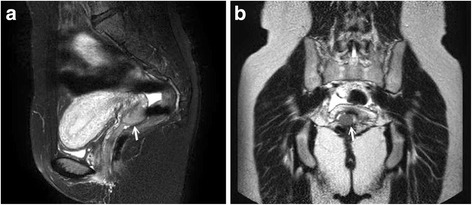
Pelvic magnetic resonance imaging findings prior to neoadjuvant chemotherapy. The lesion measured approximately 2.7 × 1.8 × 1.5 cm and was located over the right side of the cervix. The tumor was confined to the cervix, with no invasion to the adjacent tissue, parametria, or vagina. a A sagittal plane, T2-weighted image shows hyperintensity with fat saturation and gadolinium enhancement. The repetition time (TR) was 2966, and the echo time (TE) was 70. b A coronal view T2-weighted image shows an intermediate signal lesion, with a TR of 692 and a TE of 150
